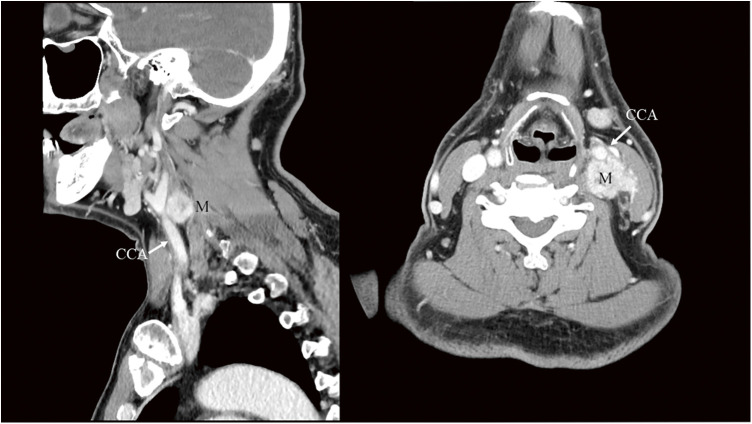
Fig. 1 Sagittal and axial planes of preoperative enhanced computed tomography. M: left cervical mass; CCA: left common carotid artery
